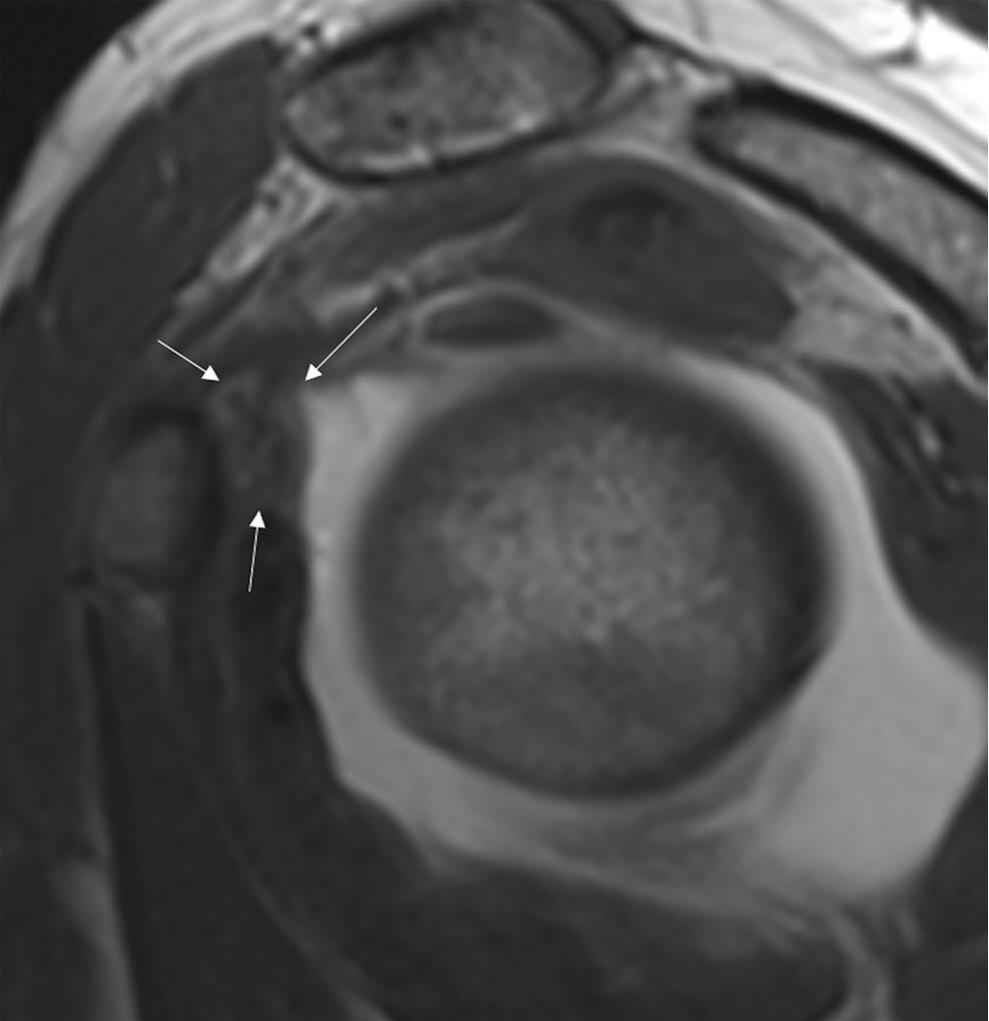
MR arthrography, sagittal oblique T1-weighted image. In a patient with adhesive capsulitis for 15 weeks, the fat triangle (*arrowheads*) signal is considerably reduced
